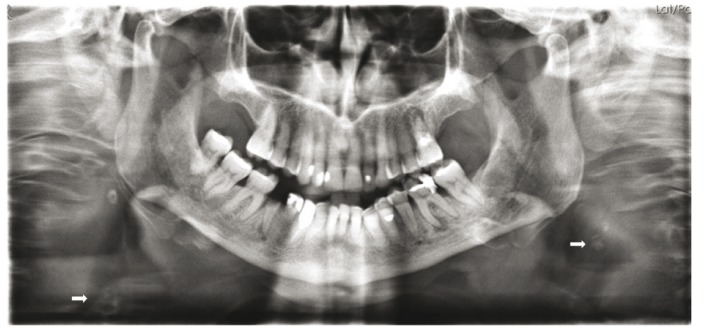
Orthopantomograph showing presence of carotid calcification on right side.
